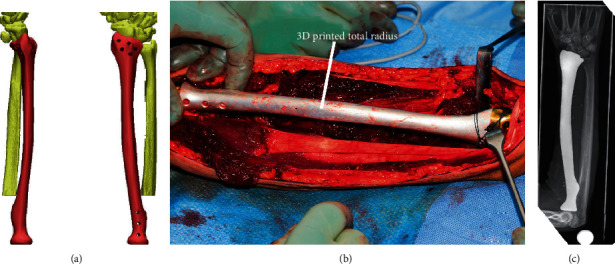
A 53-year-old man with a dedifferentiated leiomyosarcoma of the radius. (a) Virtual three-dimensional model of the radius. (b) Reconstruction with the 3D printed total radius. (c) X-ray image almost one year after implantation.
